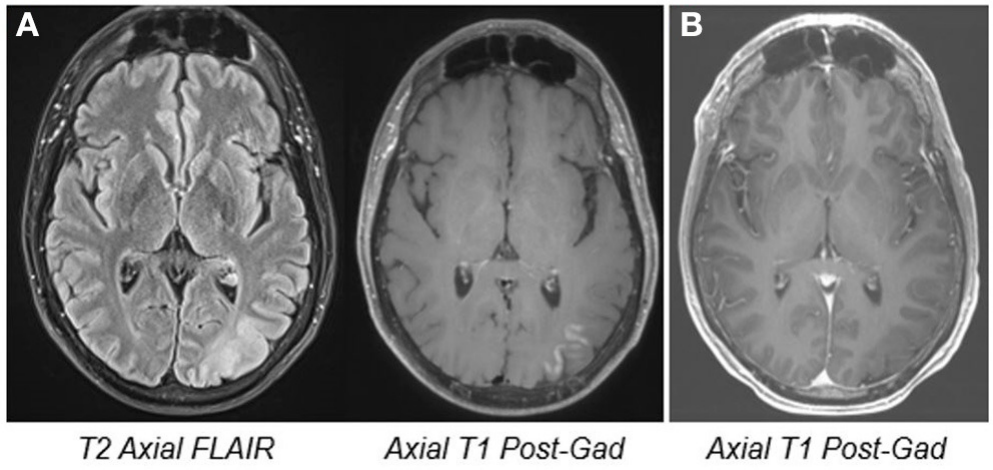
MRI images. Pre- (A) and 6 month post-treatment MRI (B) showing left occipital cortical thickening and blurring of gray-white junction, implying the presence of a focal cortical dysplasia. The pre-treatment cortical enhancement resolved at the follow-up study.
